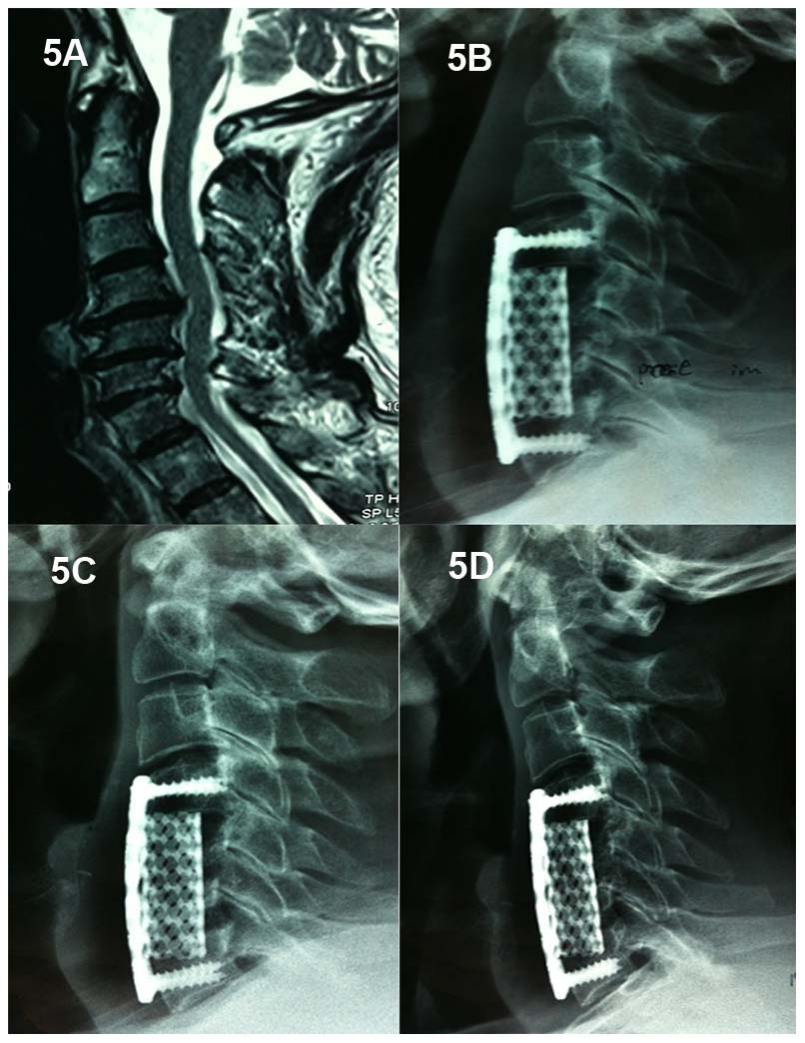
A 46-year-old male who underwent 2-level corpectomy with a titanium mesh cage used for cervical reconstruction. A cervical MRI scan (5A) shows multi-level disc herniations (C4/5, C5/6, C6/7) and oppression of the spinal cord. The immediately postoperative lateral X-ray (5B) shows C5 and C6 corpectomy and the titanium mesh cage used for reconstruction in which the cervical alignment was marginally restored. A lateral X-ray at the one-year follow-up (5C) shows subsidence. A lateral X-ray at the final follow-up (5D) shows increased subsidence and a loss of cervical alignment.
